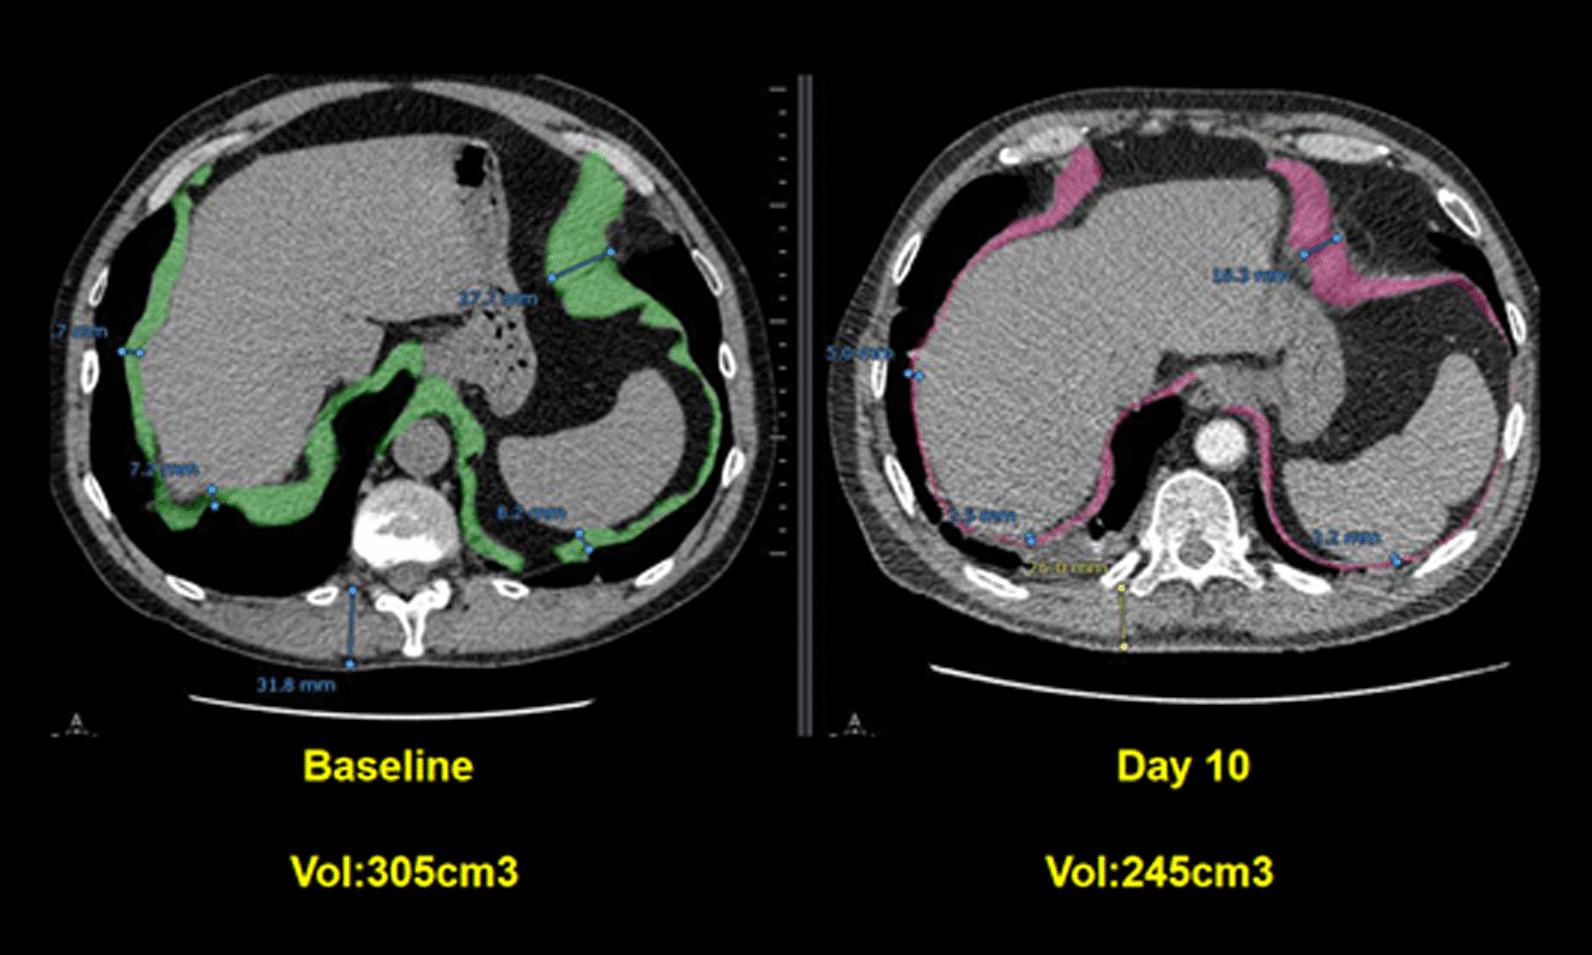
Computed tomography measurements of diaphragm volume in a critically ill, septic patient on admission to the intensive care unit (*left panel*) and ten days later (*right panel*). Sepsis was associated with a decrease in diaphragm volume. The images were provided, with permission, by Drs. Boris Jung, Stephanie Nougaret and Samir Jaber, University Hospital of Montpellier, France
